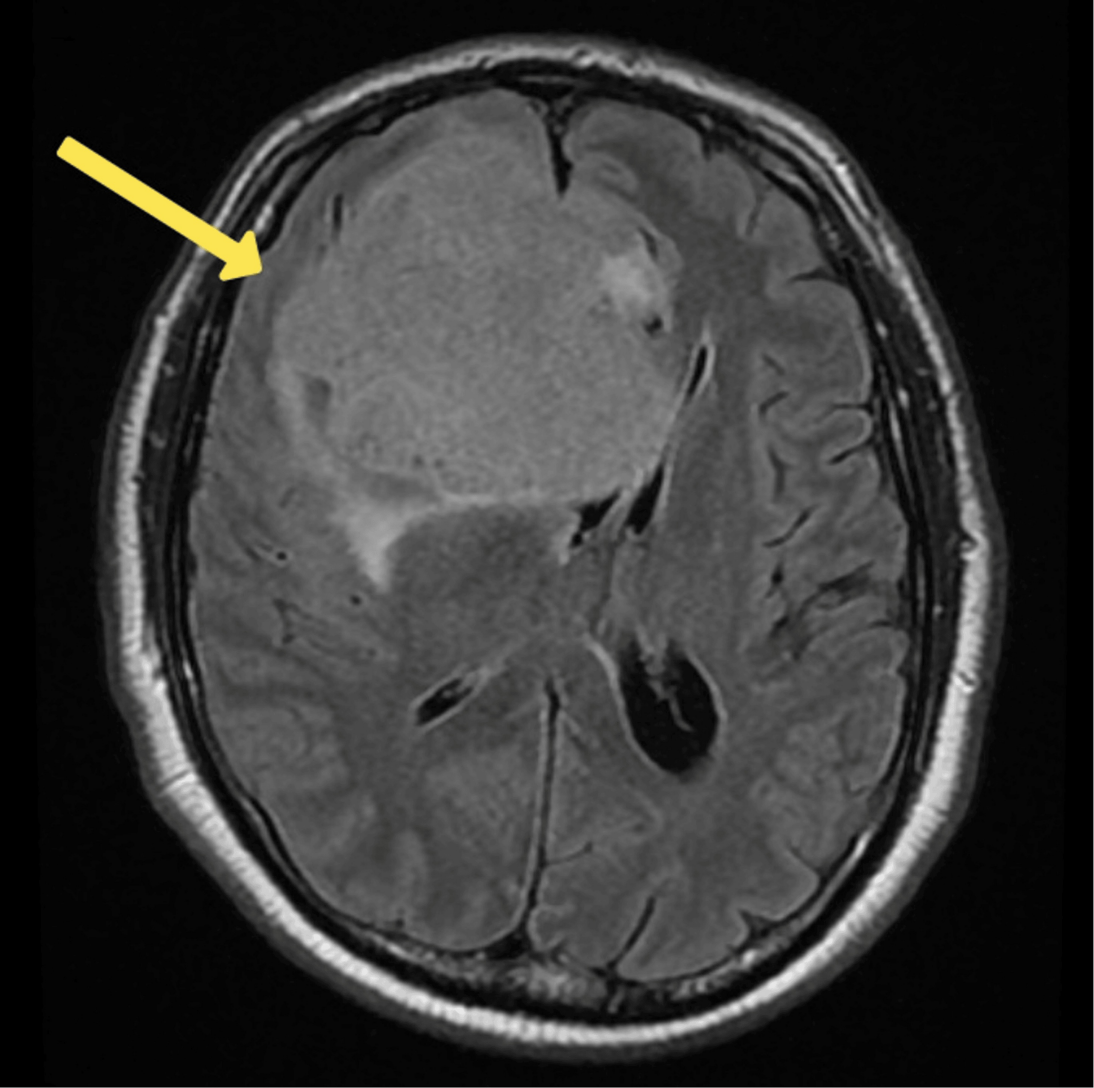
MRI brain reveals a large extra-axial mass (measuring approximately 7.1 x 7.7 x 7.5 cm) in the anterior cranial fossa on the right side (with associated mass effect and perilesional edema), causing erosion of the right anterior clinoid process, the roof and lateral wall of the right sphenoid sinus, and the superomedial wall of the apex of right orbit. The mass is seen to cause a mass effect, as evidenced by the midline shift towards the opposite side and compression of the ipsilateral lateral ventricle. The brain parenchyma is compressed and shifted superiorly. Impression: likely meningioma (see arrow).
